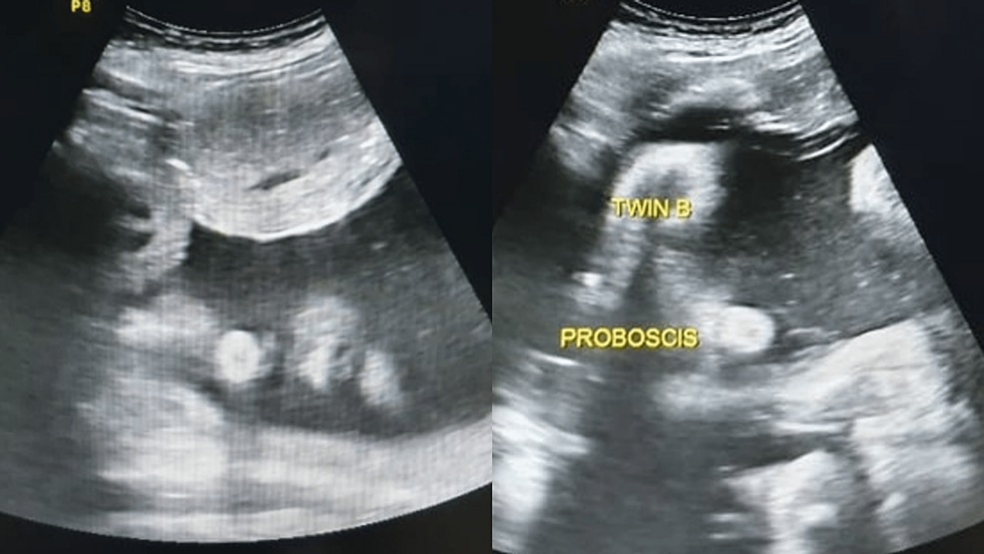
Ultrasound images of the twins at 35 weeks. The first twin is on the left, both twins show proboscis
